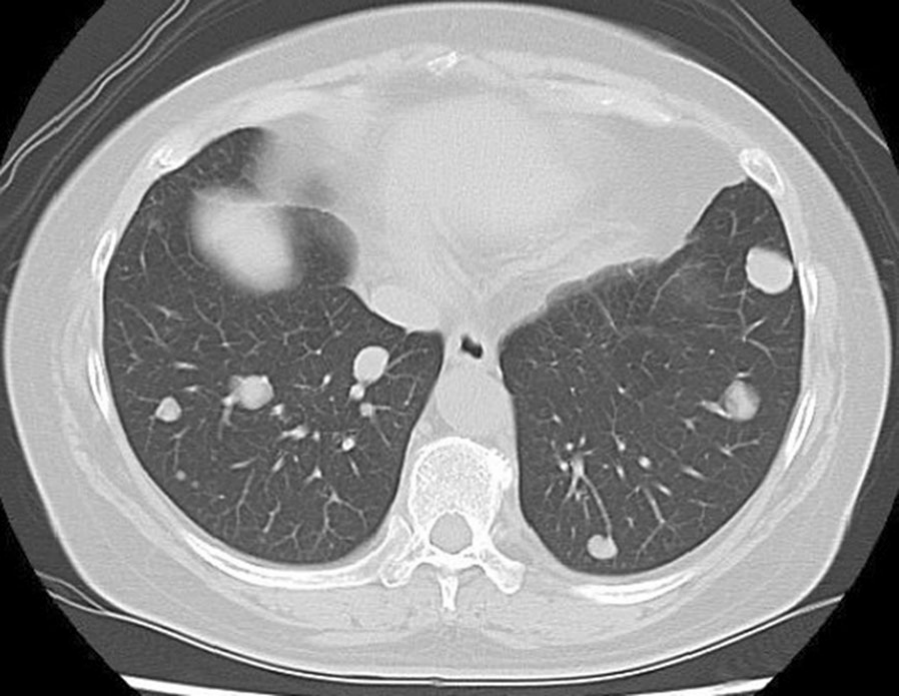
Lung CT findings: the CT images also showed multiple metastatic lung tumors
